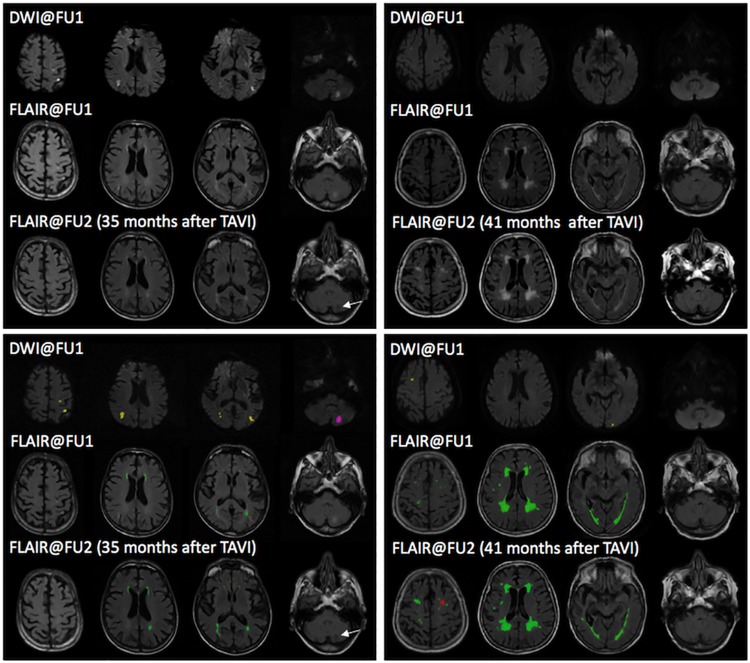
Representative MRIs of patients after TAVI. Above: Representative MRI data sets of two patients showing raw data (upper row) and overlay images (lower row). Images were acquired early (DWI@FU1 and FLAIR@FU1) and late (FLAIR@FU2) after TAVI. Below: On overlay images, yellow lesions display acute procedural CVEs which vanished without detectable remnants @FU2, purple lesions display acute procedural DW-CVEs with scarring @FU2, red lesions display “new”, non-procedural SBIs @FU2 in a location where previously no DW-CVEs were present, and green lesions display WMHs. DWI@FU1 after TAVI shows procedural CVEs in both patients. FLAIR imaging acquired at FU1 shows mild (left) and severe (right) WMH-load. However, both demonstrated only slight increase throughout the follow-up period of 35 and 41 months, respectively. The left patient developed a parenchymal defect in the left cerebellum (FLAIR@FU2) in the same location of the DW-CVE at FU1, indicating scarring of a procedural CVE. In contrast, the right patient developed an infarct-like lesion in the left frontal lobe in a location where no DW-CVE was previously recognized at FU1 (“new”, non-procedural SBI). Only a fraction of peri-procedural, acute DW-CVEs resulted in detectable infarct-like lesions in follow-up scans (arrow). DWI–Diffusion-weighted imaging, CVE–Cerebrovascular event, WMH–White matter hyperintensities, SBI–Silent brain infarction, FLAIR—Fluid Attenuated Inversion Recovery, FU–Follow-up, TAVI–Transcatheter aortic valve implantation.
